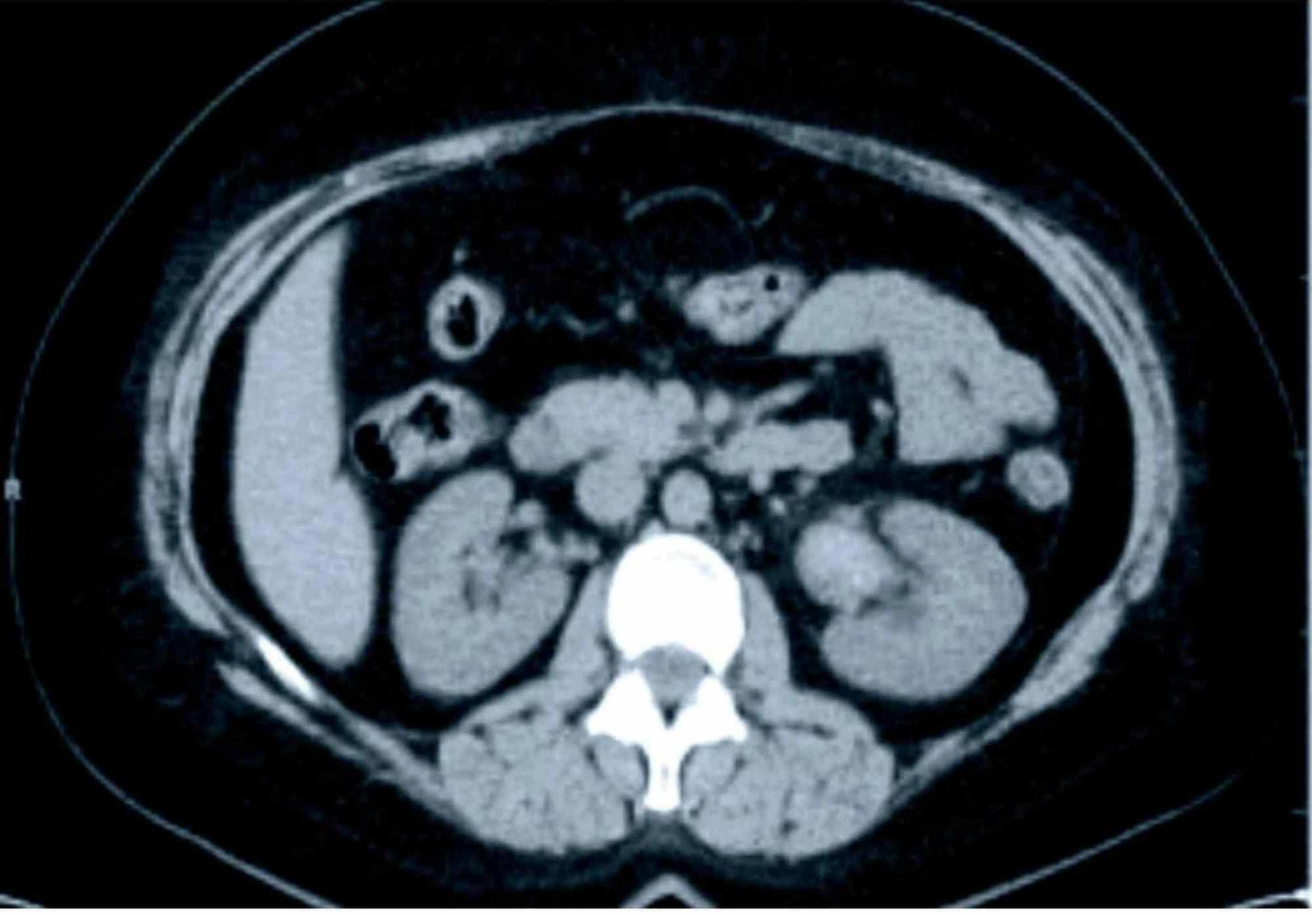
CT scan of the abdomen showing bilateral nephromegaly.
